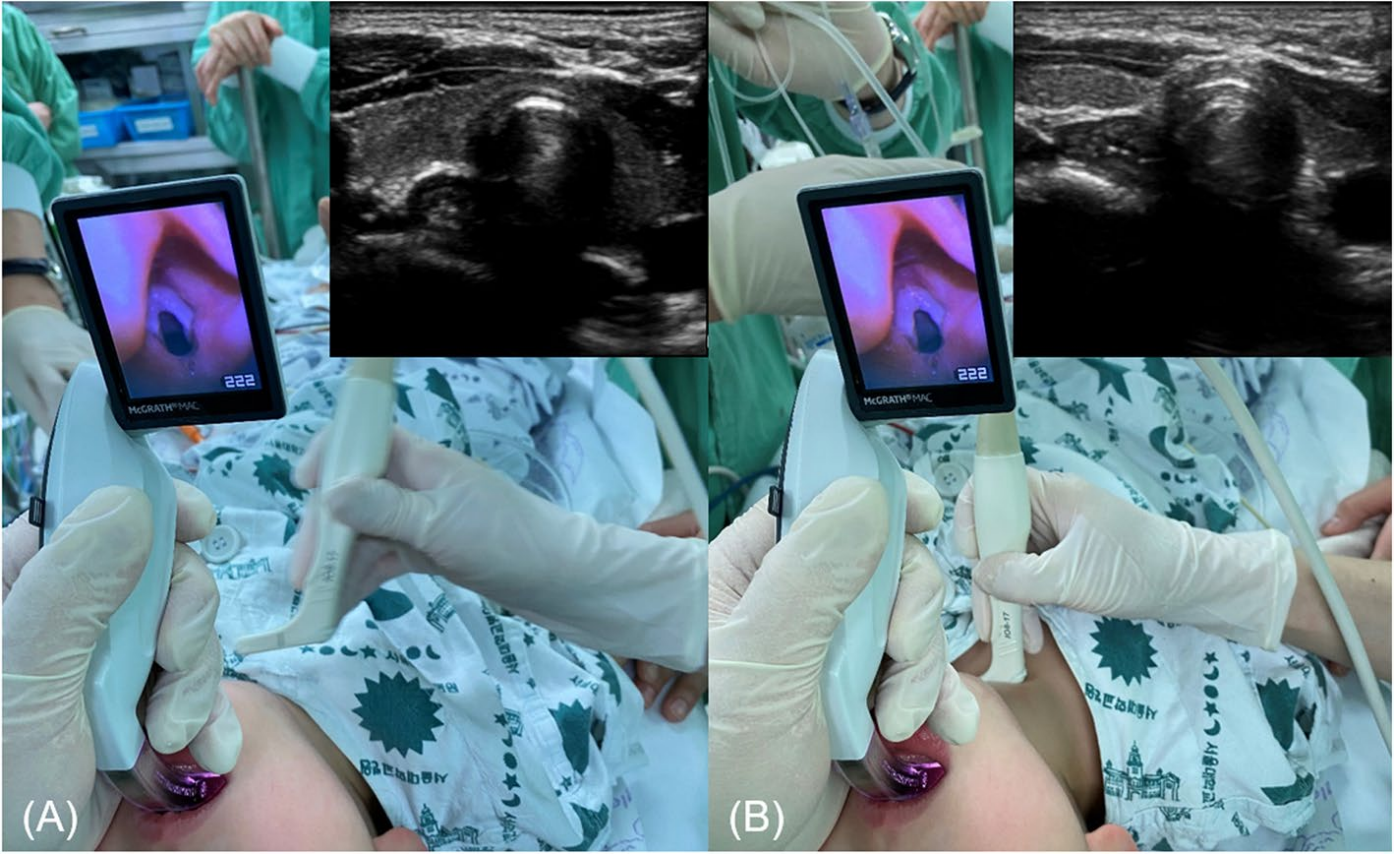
Glottic opening score percentages before (A) and after (B) ultrasound-guided esophageal compression was applied in a boy aged 23 months who weighed 12.5 kg. Before (A) and after (B) ultrasound-guided esophageal pressure application in a girl aged 14 months and weighing 12.2 kg
